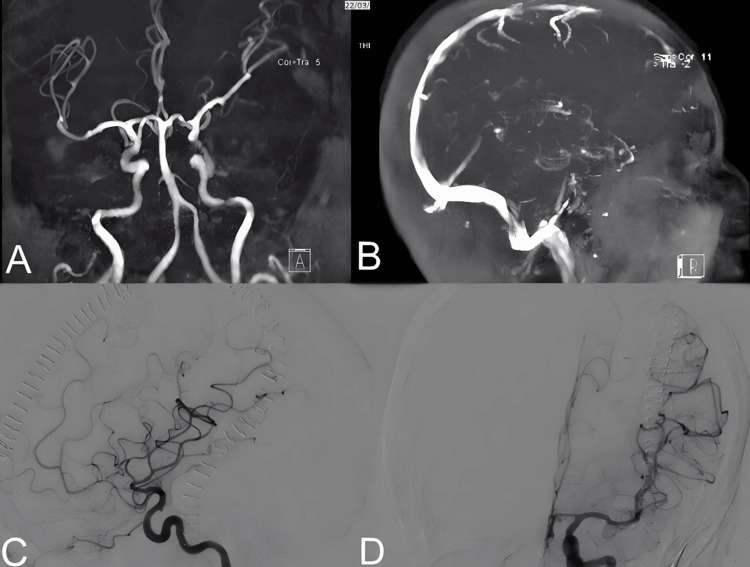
Brain MRA and MRV A: Brain MRA. B: Brain MRV. C: Lateral view DSA. D: Anteroposterior (AP) view DSA showing no evidence of any aneurysms, AVM, fistula, or other vascular pathologies. SICH: Spontaneous Intracranial Hemorrhage; DSA: Digital Subtraction Angiography; FLAIR: Fluid Attenuated Inversion Recovery; MRA: Magnetic Resonance Arteriography; MRV: Magnetic Resonance Venography; AVM: Arteriovenous Malformation
